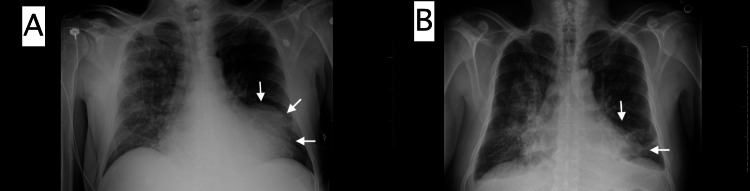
Chest radiographs A: Chest radiograph with new left ventricle border configuration (white arrow). B: Previous chest radiograph with original left ventricle border configuration (white arrow).
